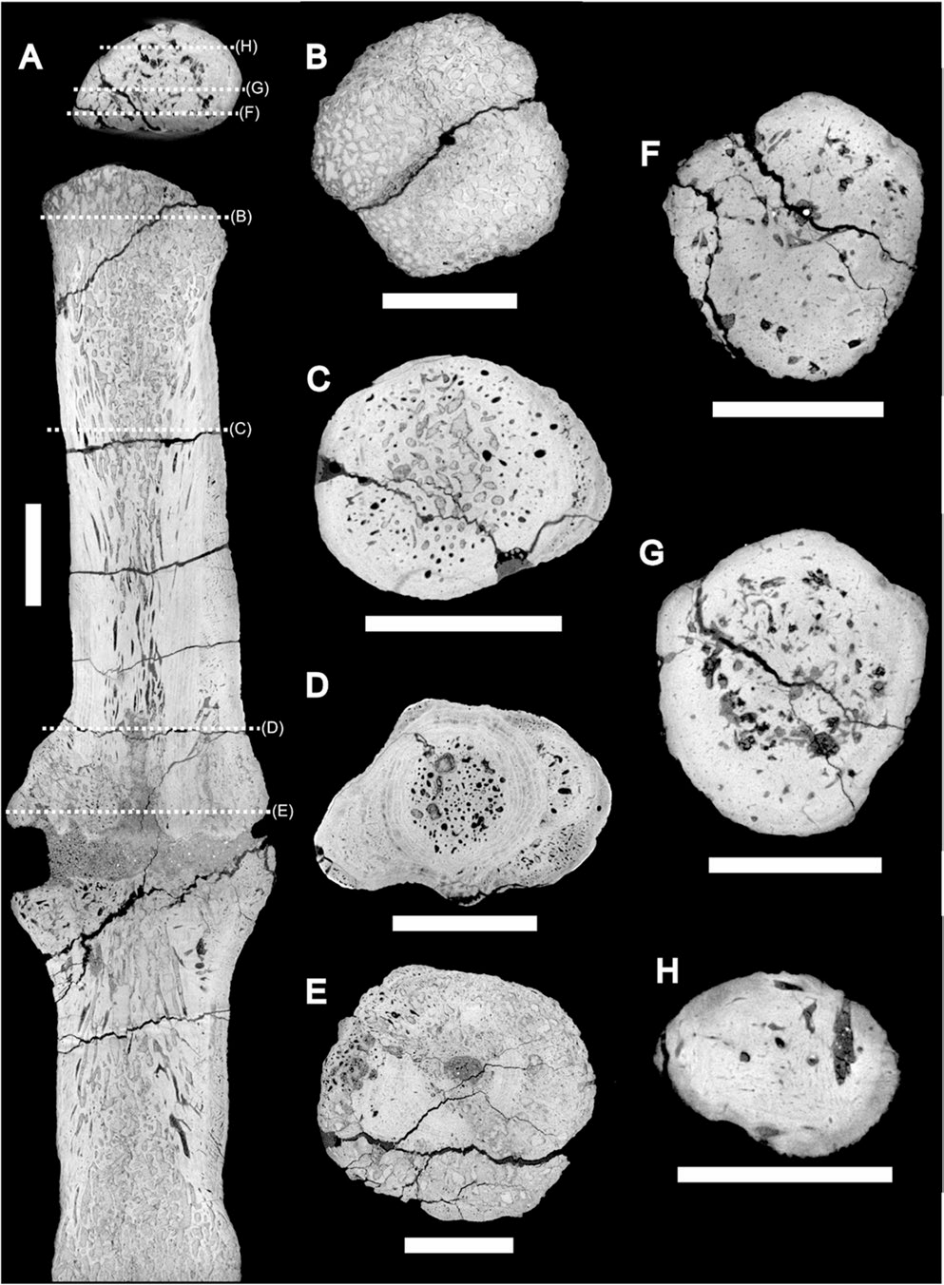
CT sections of CdA and CdB. Sagittal section (A) roughly at midheight of CdA and CdB, showing the vascularization and ‘chaotic’ growth near the callus, as well as the sediment dividing the callus. Transverse sections of CdA show the transition from near the anterior articular surface (B), normal vertebral bone (C), clear periosteal callus formation (D), to inside the pathological callus (E). Transverse sections of CdB show the transition from near the articular surface (F), the middle of the vertebra (G), to the posterior rim of CdB. Note that for scanning purposes, CdA and CdB were scanned together, as seen in (A). All scale bars equal 1 cm
